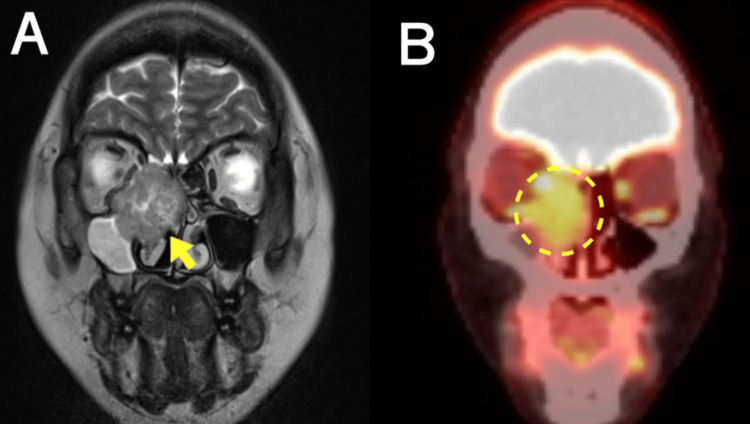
MRI and PET/CT images showing local recurrence after surgery A. Coronal T2 MRI showing an invasive tumor in the orbit and adjacent to the skull base (arrow). B. PET/CT demonstrating elevated FDG uptake in the recurrent tumor (dashed line). MRI, magnetic resonance imaging; PET/CT, positron emission tomography/ computed tomography; FDG, fluorodeoxyglucose
